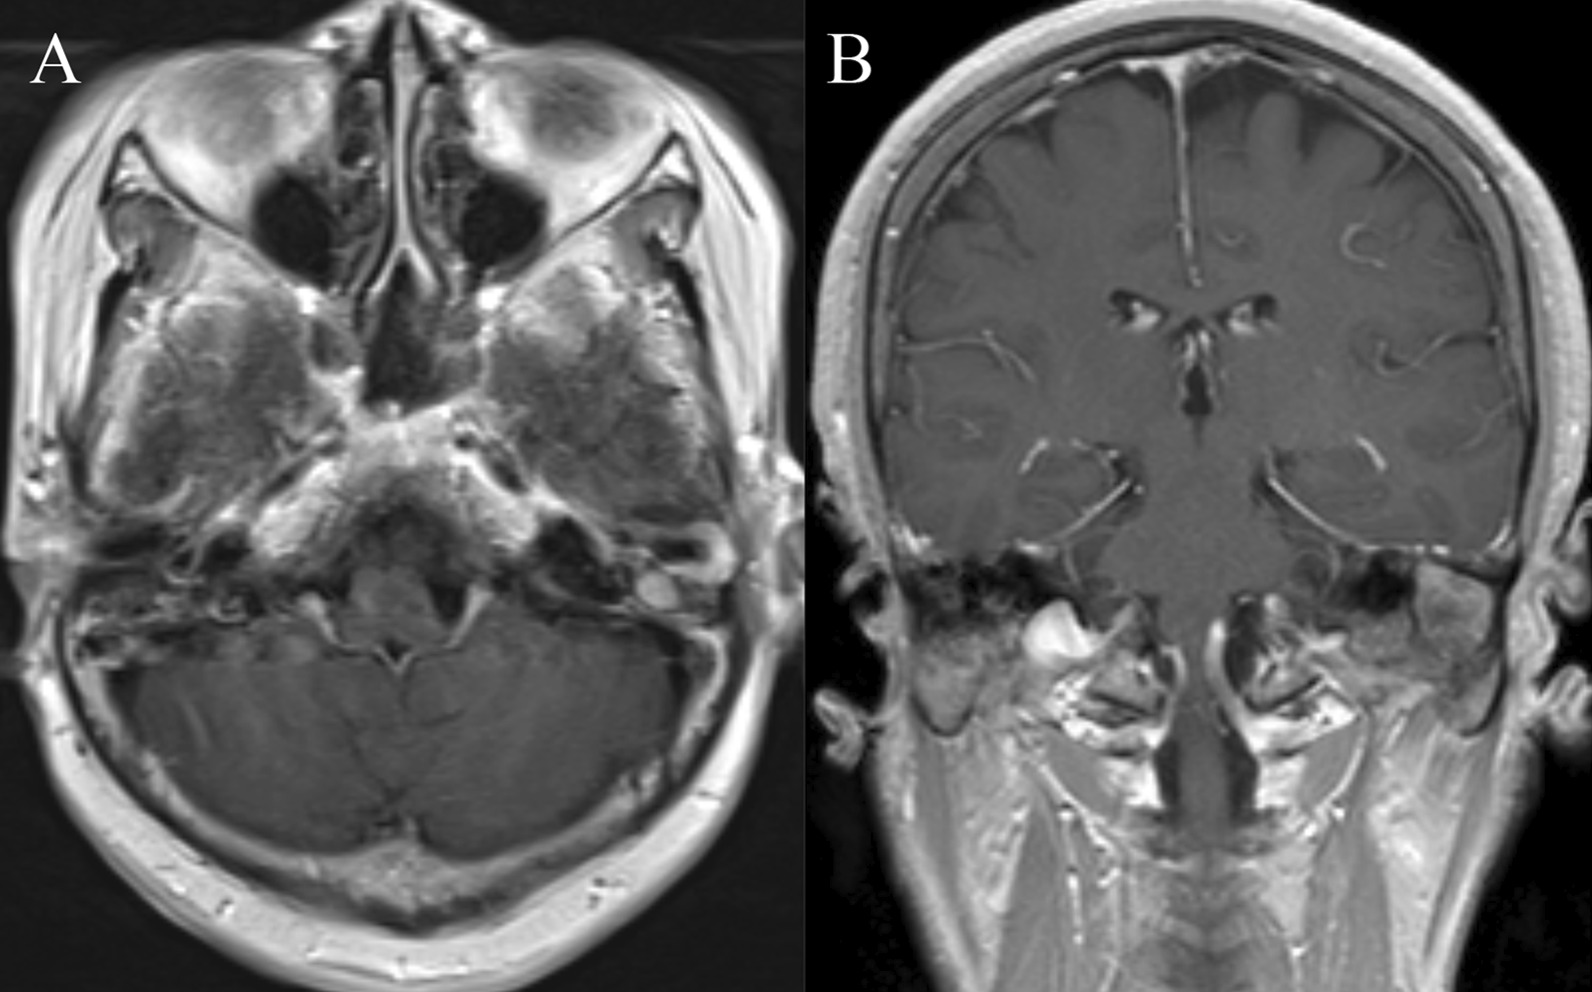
Magnetic resonance imaging revealed structures of the tumour
